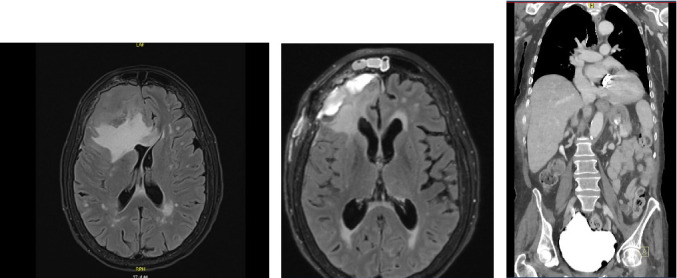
(a and b) Axial T2-FLAIR MRI showing right frontal extra-axial mass (8 × 6 × 6 cm) with midline shift. (c) CT abdomen shows no hepatosplenomegaly; the liver appears prominent, but no enlargement was measured.
